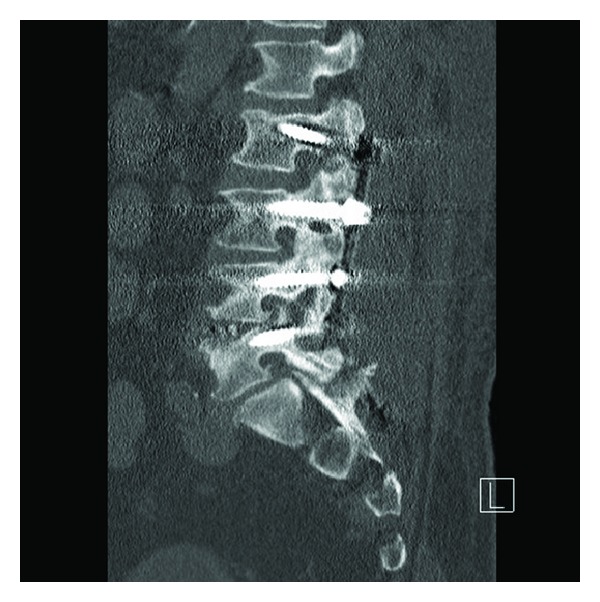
Sagittal view of CT scan showing loose L2 screws and a pseudarthrosis at the L2-L3 level.
